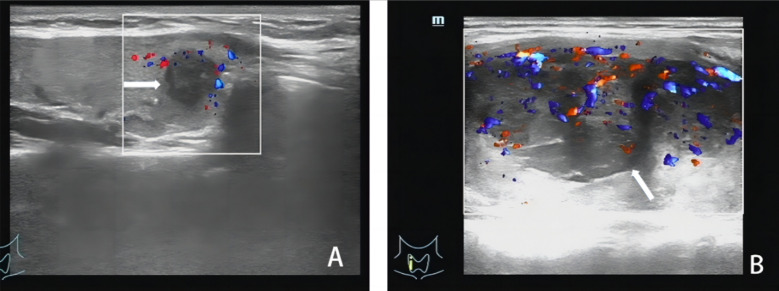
Ultrasound examination images of different stages of thyroid malignant tumors. A: Low velocity blood flow in stage II patients; B: High blood flow in stage IV patients.
